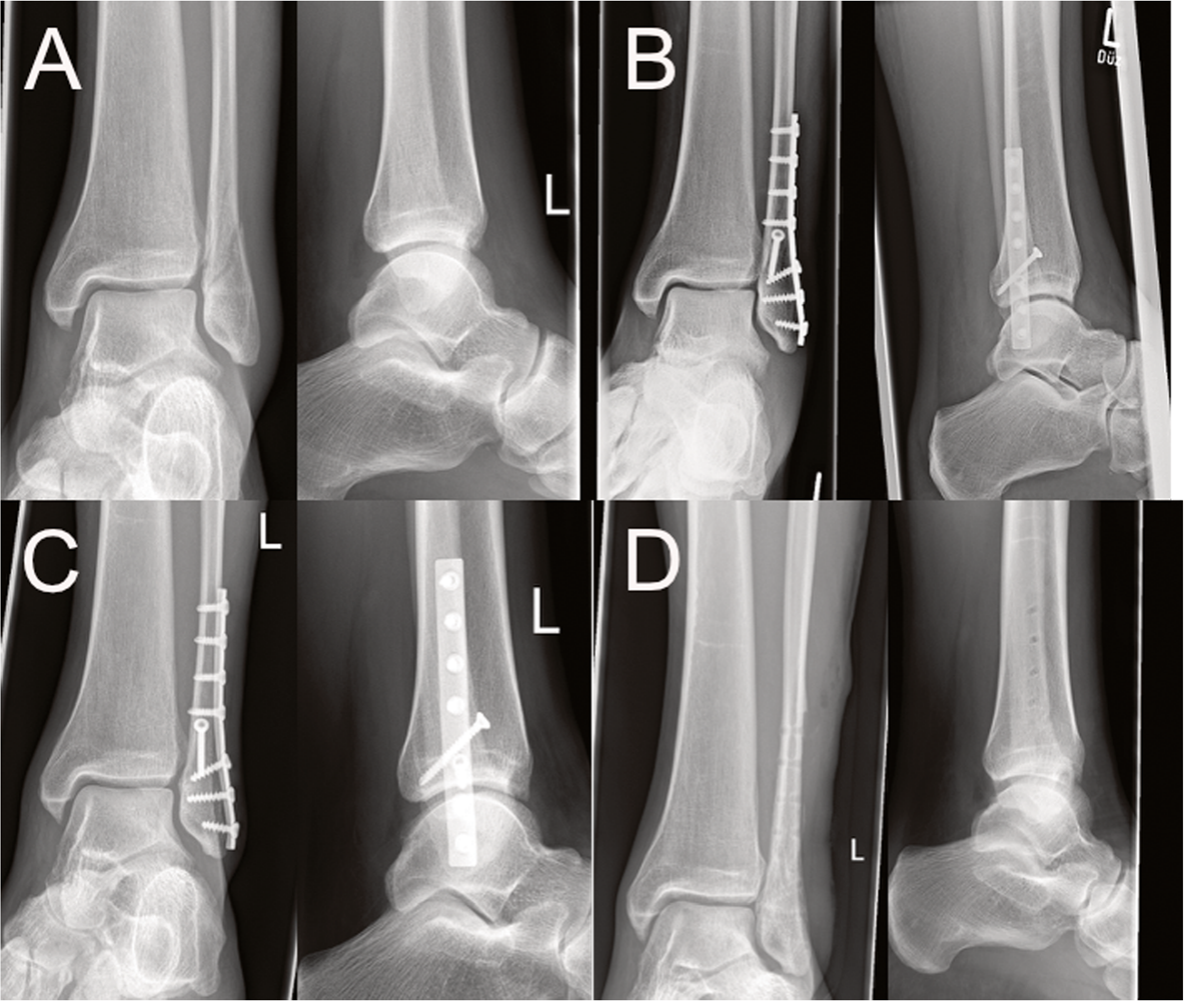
Radiographs in two planes of a 38-year-old male patient with an AO type 44B1.1 fracture (a) treated with the DePuy Synthes® one-third semi tubular plate system. Six weeks (b) and 12 months (c) postoperative follow-up radiographs as well as the control after hardware removal (12 months postop, d) are shown as well
